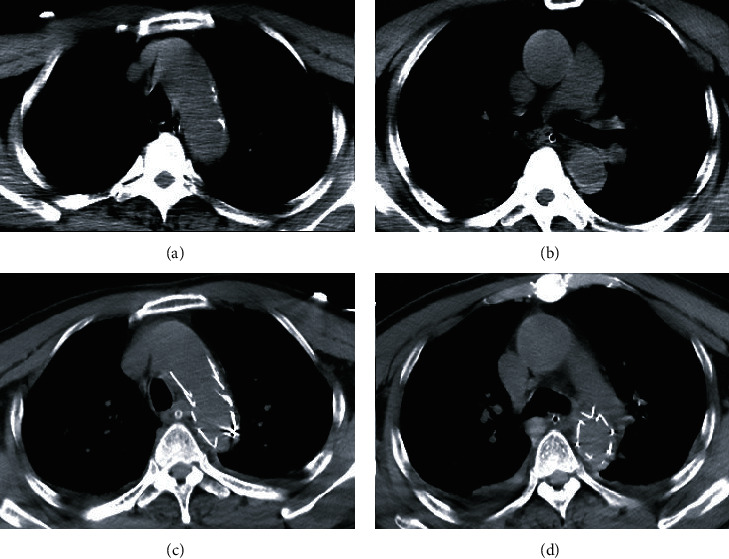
(a and b) CT angiography scan showed a suspicious crescent-shaped high-density shadow of the descending aorta. (c and d) After aortic stenting, no internal leakage was visible.
